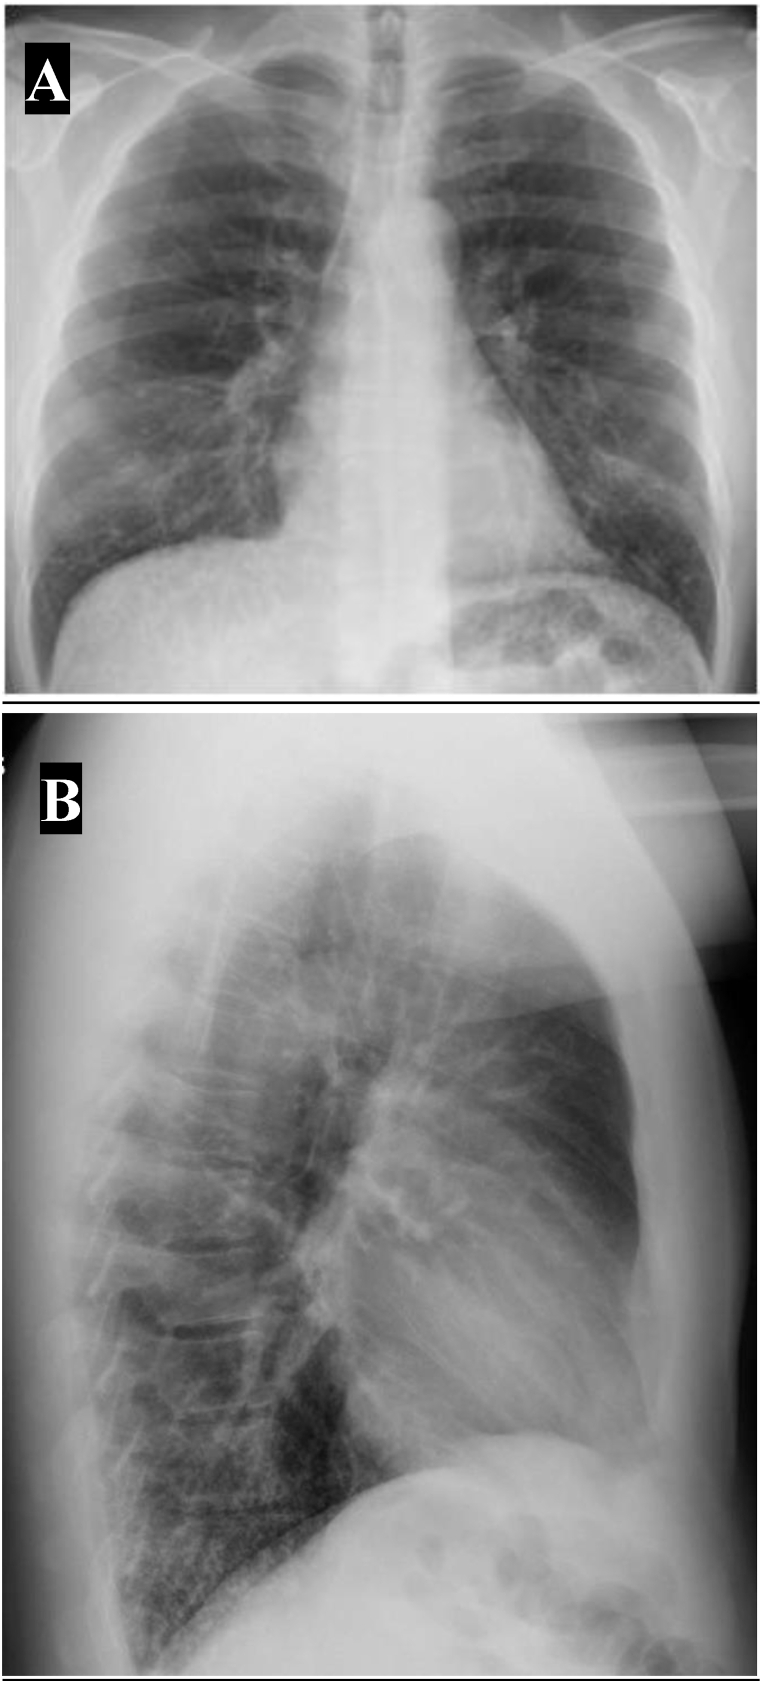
Posterior anterior (A) and lateral roentgenogram (B). Posterior basilar hyperlucent strands.
